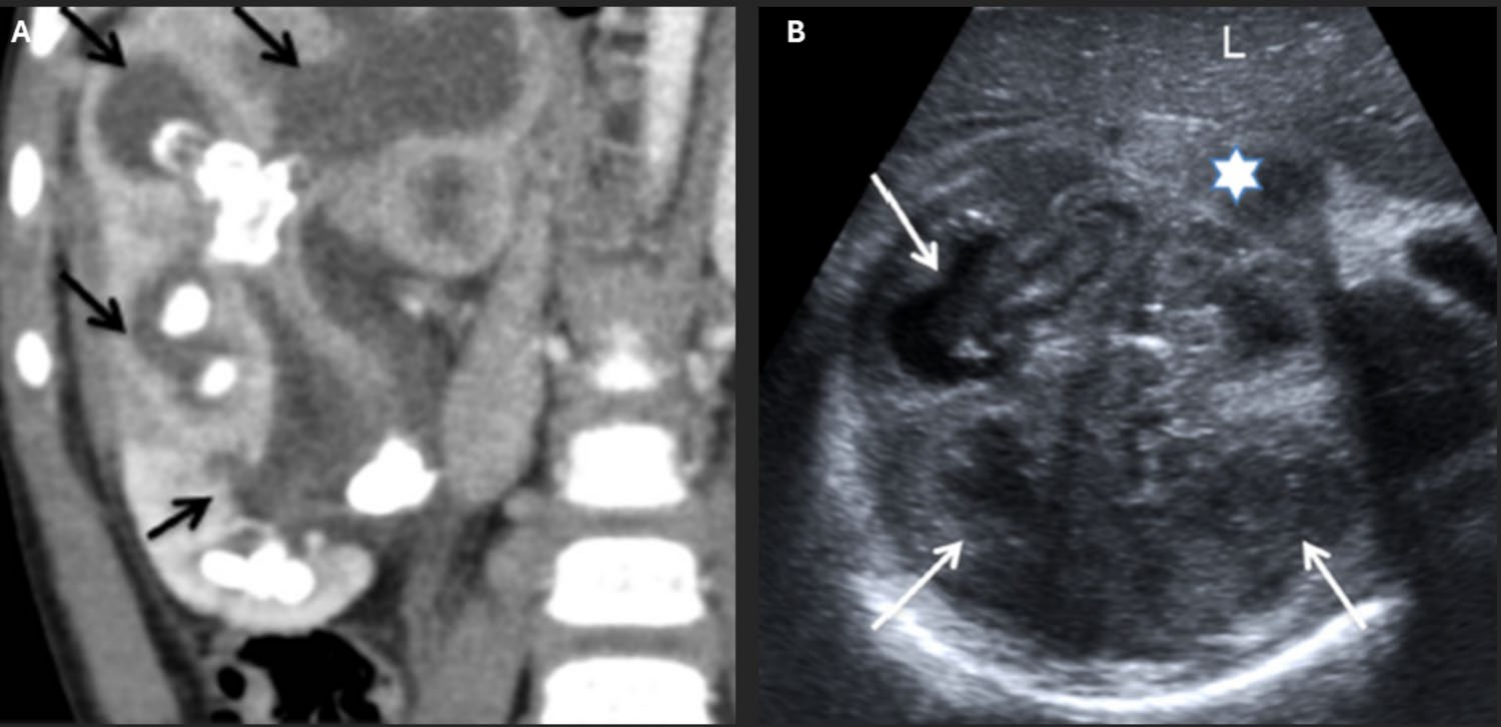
Radiological images of XPN. A Diffuse XPN. CT scan after intravenous contrast administration shows hypodense round areas (arrows) in the right kidney, accompanied by calculi in the calyces. B Localized XPN. US shows multiple hypoechoic masses (arrows) replacing normal parenchyma of the right kidney, along with the presence of perinephric fluid (star). Adapted from reference [36], reproduced under Creative Commons License CC BY. Xanthogranulomatous pyelonephritis, XPN; ultrasound, US; computed tomography, CT
